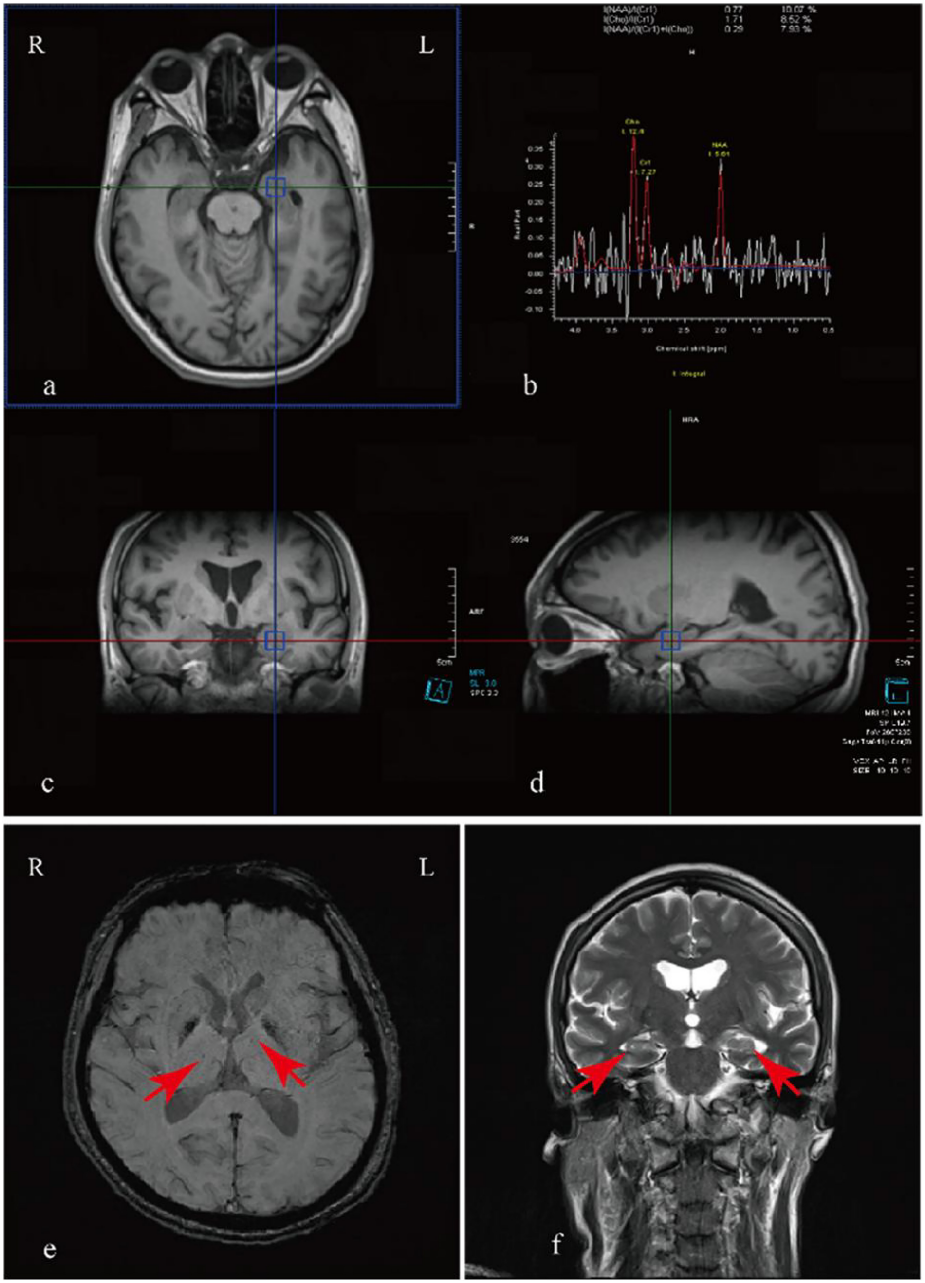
Magnetic resonance imaging findings. Cranial MRS (a–d) and MRI (f) demonstrated a reduction in the volume of the bilateral hippocampi, with more pronounced atrophy on the left side, along with widened bilateral choroidal fissures and mild dilation of the bilateral lateral ventricle horns. Cranial SWI (e) revealed minor, spotty, and patchy deposits of paramagnetic material in the bilateral basal ganglia regions.
